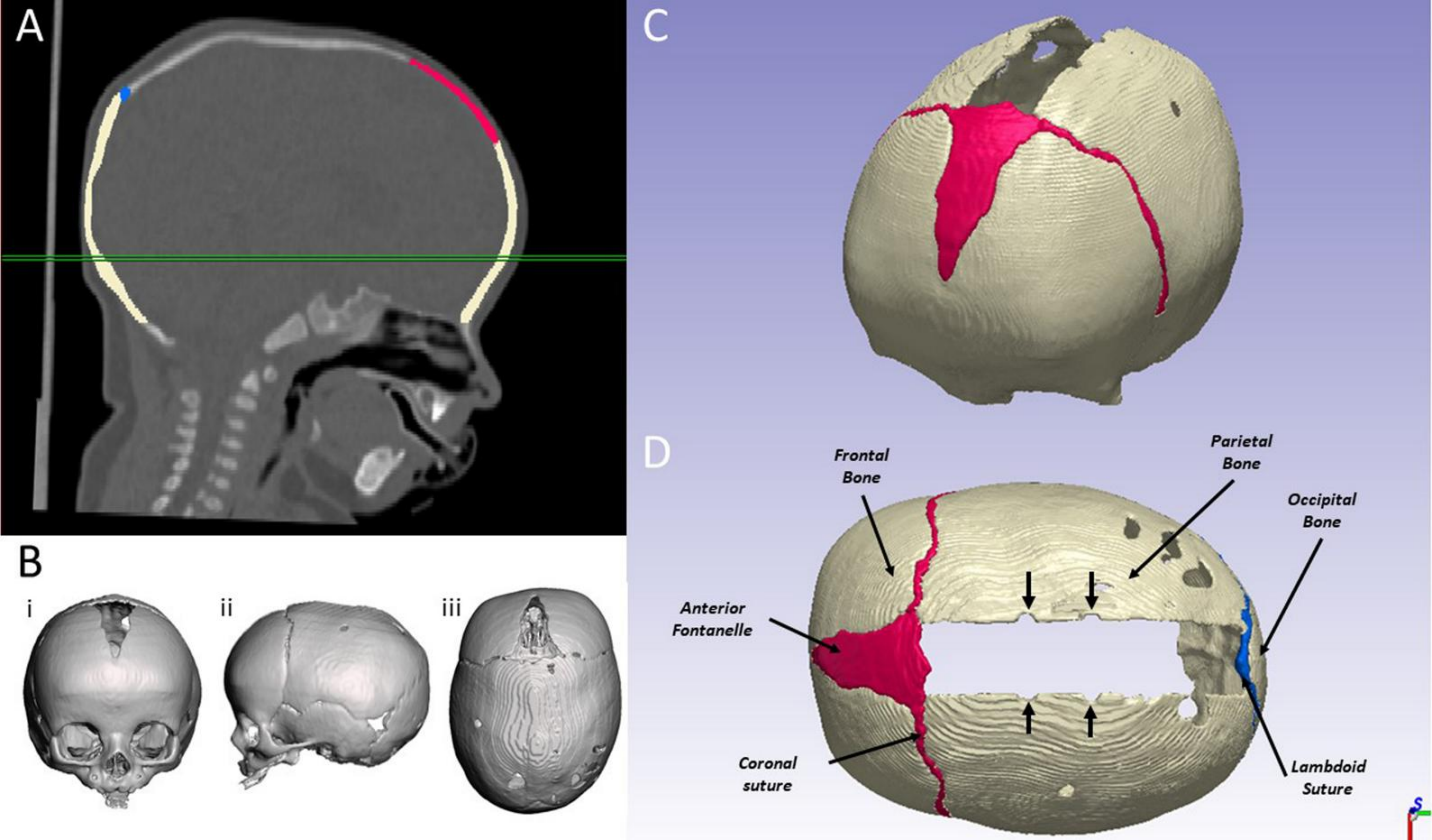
Creation of the CT model from preoperative CT scans: A) image segmentation of a representative patient, with B) front(i), side (ii) and top (iii) view of the patient skull; C) isometric and D) top view of the CT model with osteotomies and spring notches (indicated with arrows).
